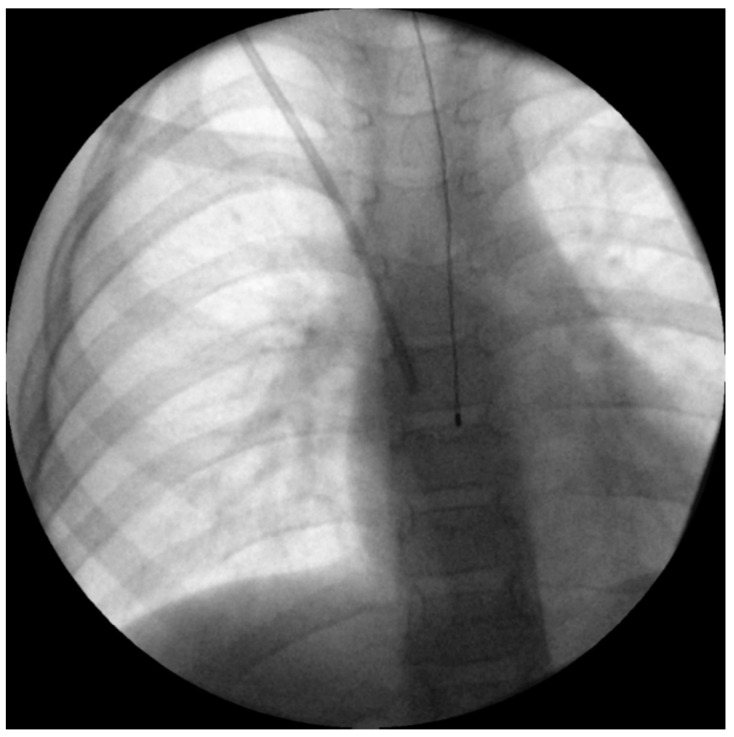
Intraoperative X-ray of a 6-year-old boy undergoing Broviac catheter implantation with appropriate depth of oesophageal temperature probe inserted, according to the estimation method by Whitby et al. [64].
